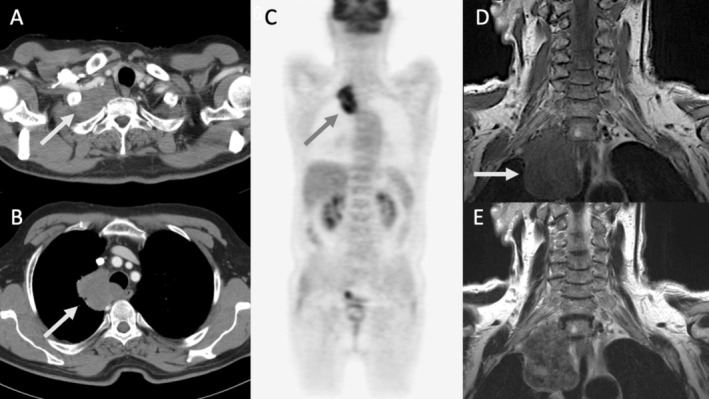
Superior sulcus (Pancoast) tumor (arrow) in a 62‐year‐old male patient. Transverse CT images (A and B) whole body PET (c) and coronal T1‐ (D) and T2‐weighted (E) MRI show tumor invasion from the right lung apex into the thoracic wall but sparing the vertebral column.
